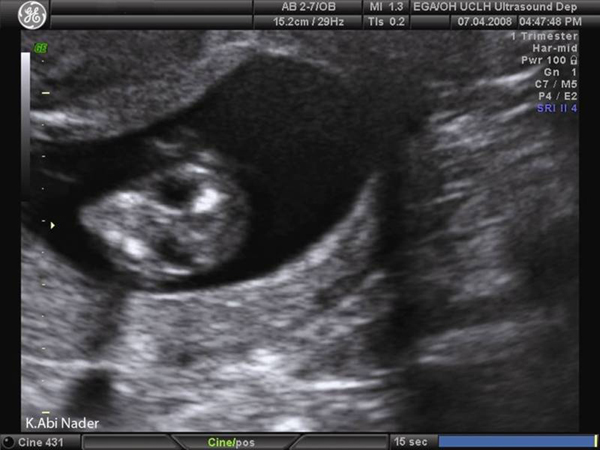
Trans-axial section: The same cysts seen in Figure 1are shown here in cross section using ultrasound. The cysts are located anterolaterally in the neck and the posterior nuchal region looks normal.
